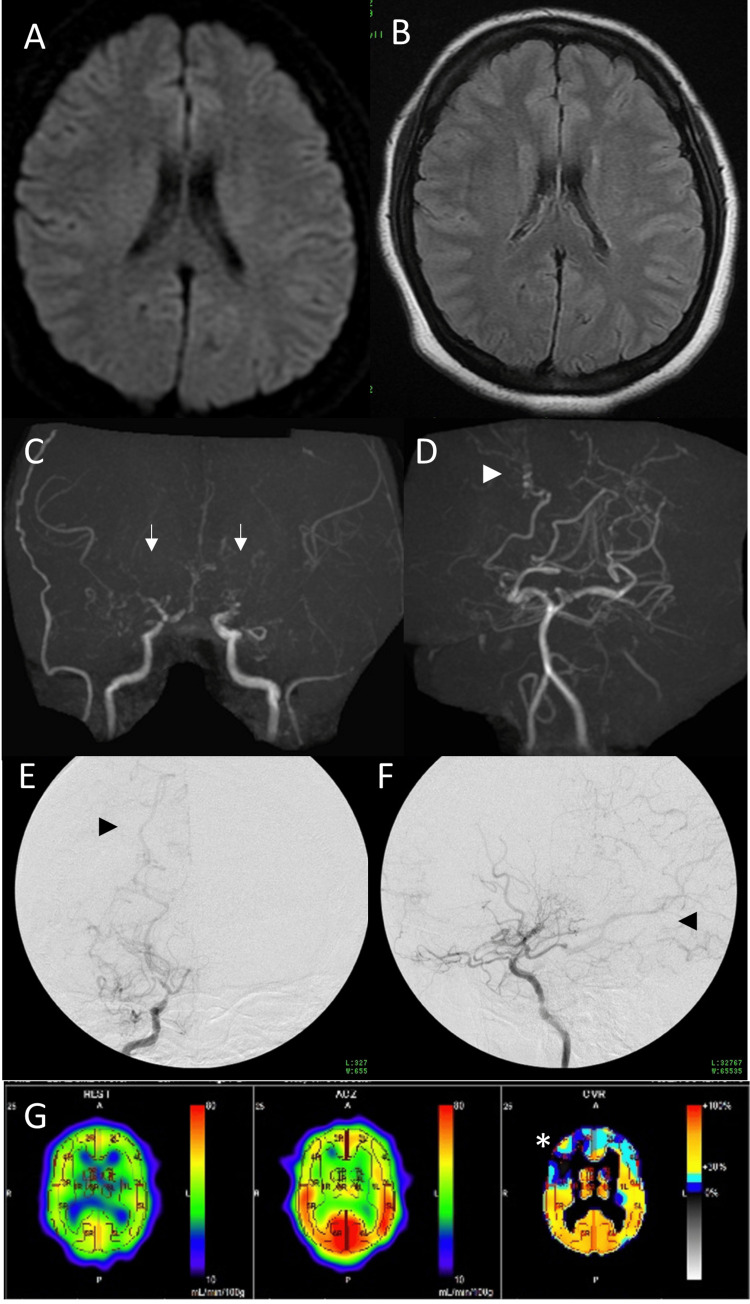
Preoperative imaging examination. A: Diffusion-weighted images showed no acute-phase infarct findings. B: Fluid-attenuated inversion recovery (FLAIR) images showed no abnormal findings; the periventricular flow void findings were unclear. C: Magnetic resonance angiography of the anterior circulation showed bilateral severe stenosis from the terminals of the internal carotid artery to the proximal portion of the anterior cerebral artery and middle cerebral artery, with nearby moyamoya vessels. D: Magnetic resonance angiography of the posterior circulation showed bilateral posterior cerebral arteries with compensatory development, but stenosis proximal to them was unclear. E and F: Preoperative digital subtraction angiography of the right internal carotid artery, anteroposterior (E) and lateral (F) views showed stenosis at the terminal of the internal carotid artery, moyamoya vessels, and a compensatory developed posterior cerebral artery (black arrowhead) are confirmed. G: Single photon emission computed tomography (SPECT) scan showed decreased resting cerebral blood flow and cerebrovascular reserve in the bilateral frontal lobes, predominantly on the right side.
